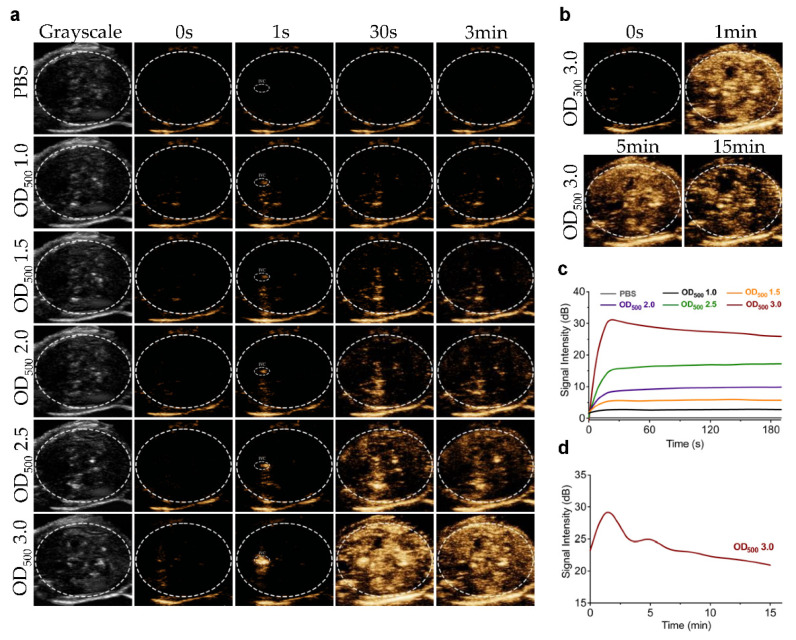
Ultrasound contrast imaging of GVs in vivo: (a) nonlinear contrast images of GVs (OD_500_ 1.0–3.0) at different time points after tail injection; (b) nonlinear contrast images of GVs at OD_500_ 3.0; (c) time–intensity curves of GVs at different concentrations in liver within 3 min; (d) time–intensity curves of GVs at OD_500_ 3.0 in liver within 15 min.
